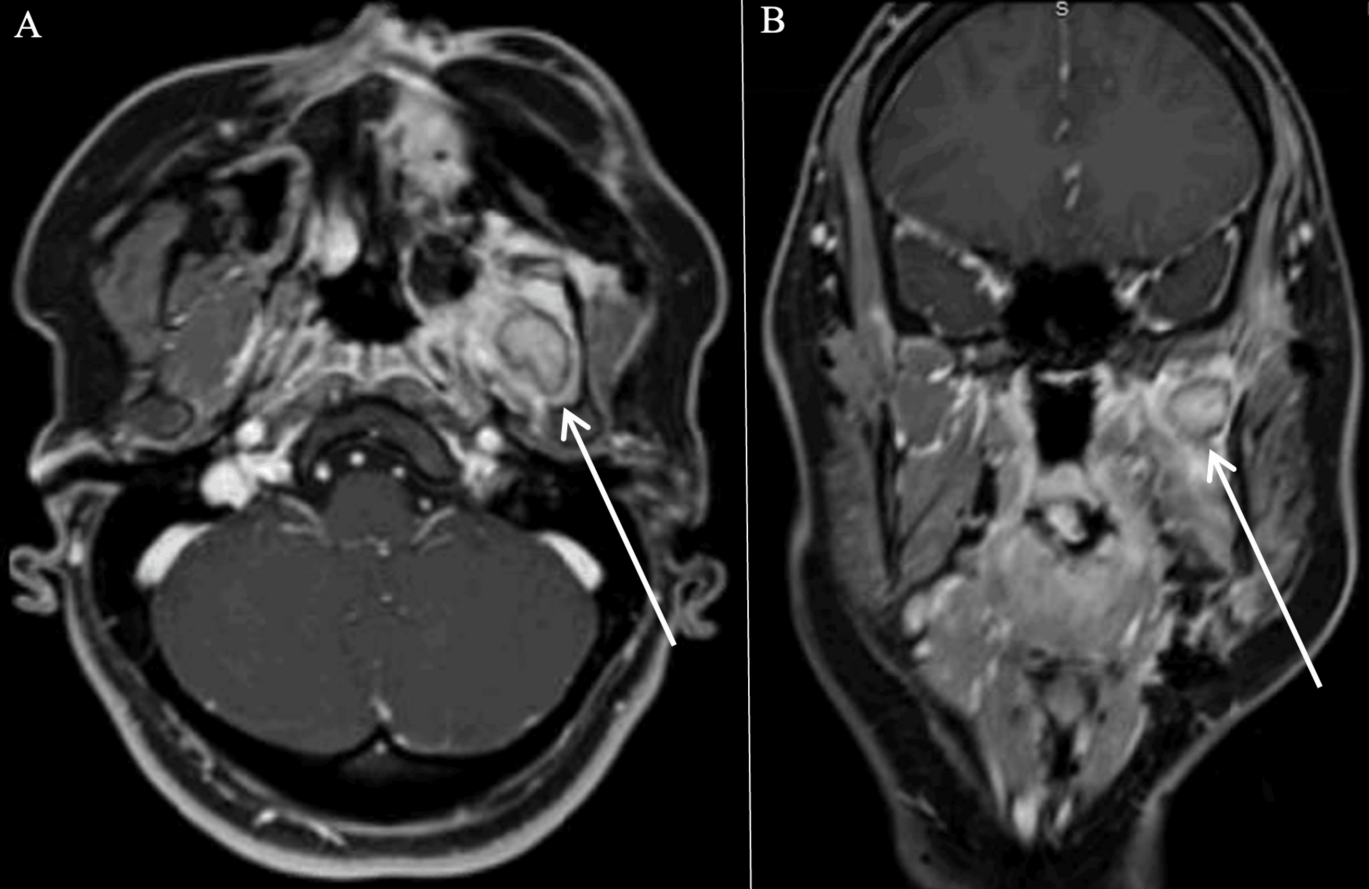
Three-month post-surgical resection imaging demonstrating a circumscribed mass within the left medial pterygoid muscle measuring 2 x 1.4 x 1.7 cm (AP x TRV x CC) with peripheral hypoenhancement on T1 axial (A) and coronal (B) MRI (face/neck/orbit) AP: anterior-posterior; TRV: transverse; CC: cranio-caudal
